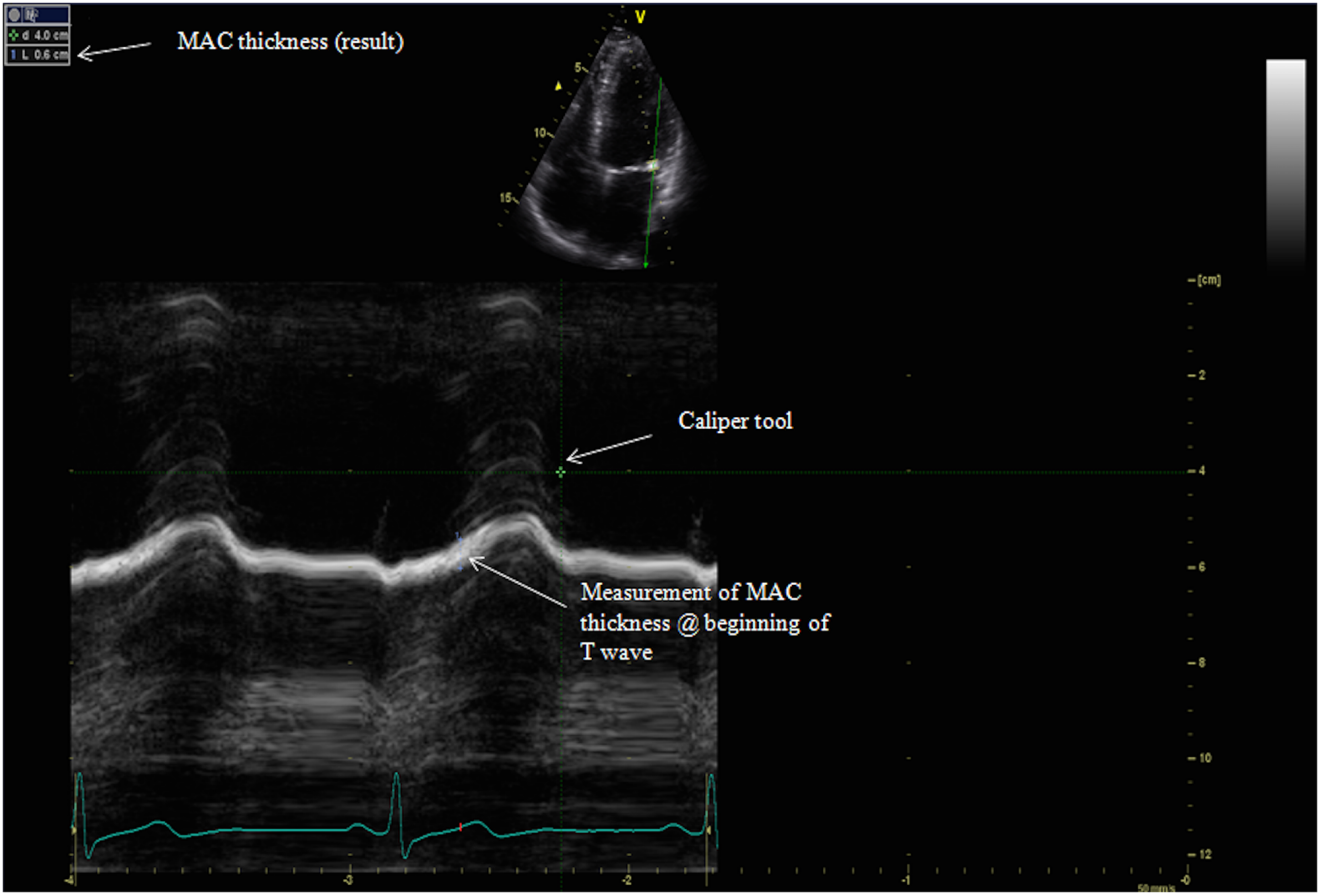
M-mode measurement of MAC in the apical four chamber view. MAC—Mitral annular calcification; cm—centimeters. Abbreviations: Mitral Annular Calcification (MAC).
